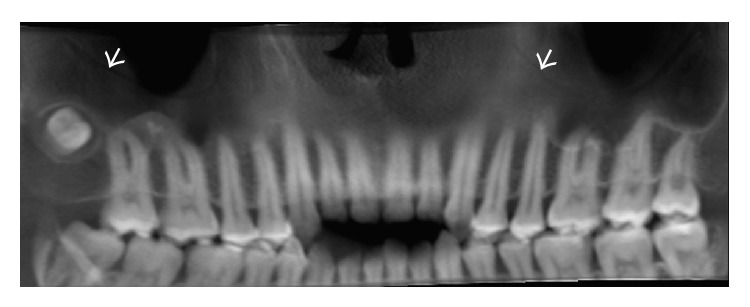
Panoramic radiograph of the bony canal pathway (white arrows).
